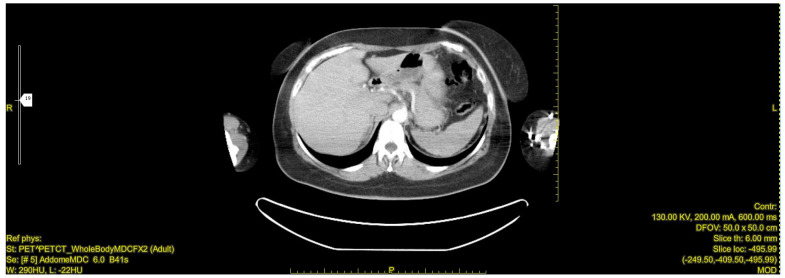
Postoperative Abdominal CT: Non postoperative complications at the surgical site.
